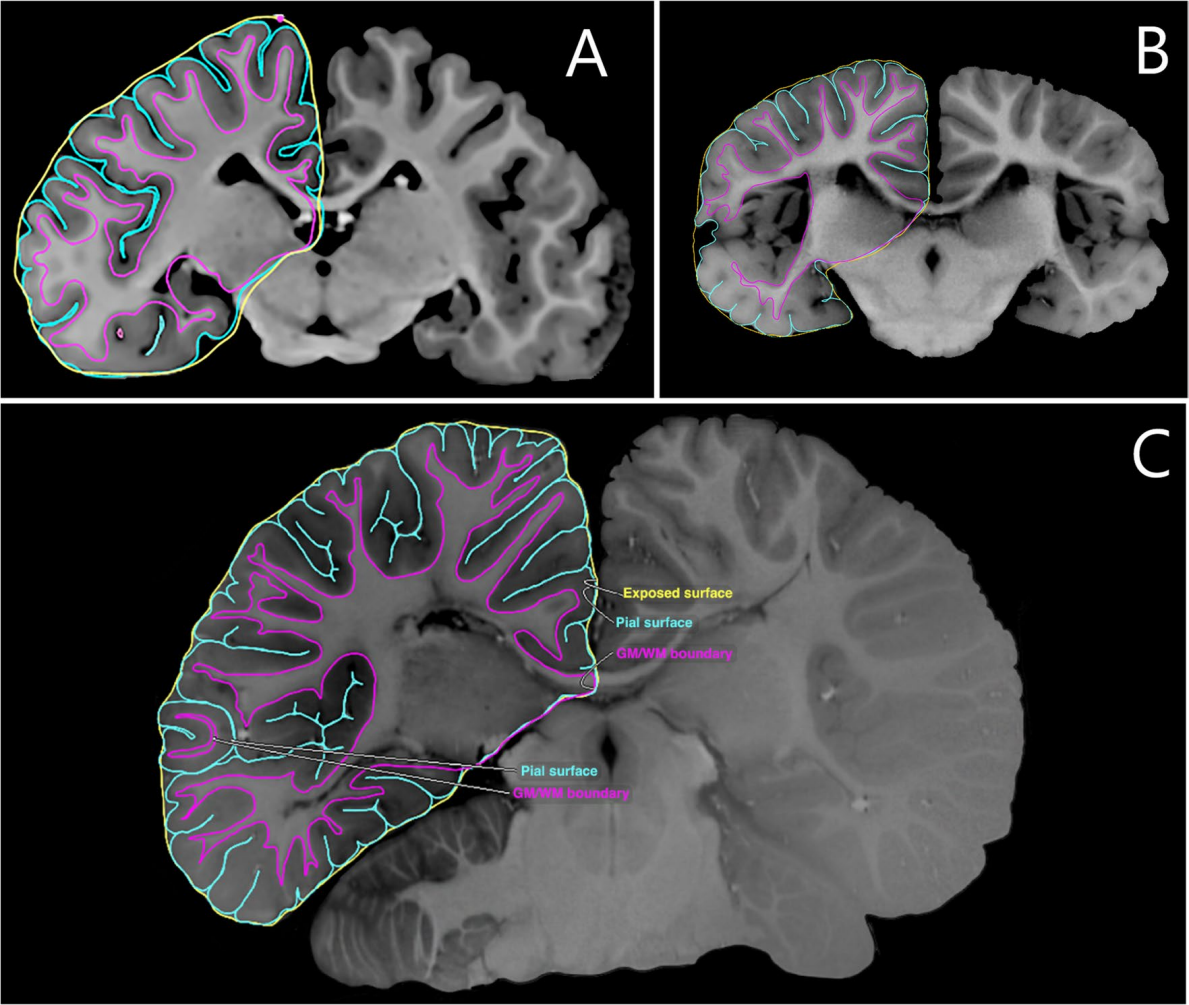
Examples of how the MRI and the manual segmentation of GM, WM and exposed surface ROIs look like for the three species in this study. A Steller sea lion. B Franciscana dolphin; adapted from Avelino-de-Souza (2023). C Guiana dolphin; adapted from Avelino-de Souza et al. (2024)
